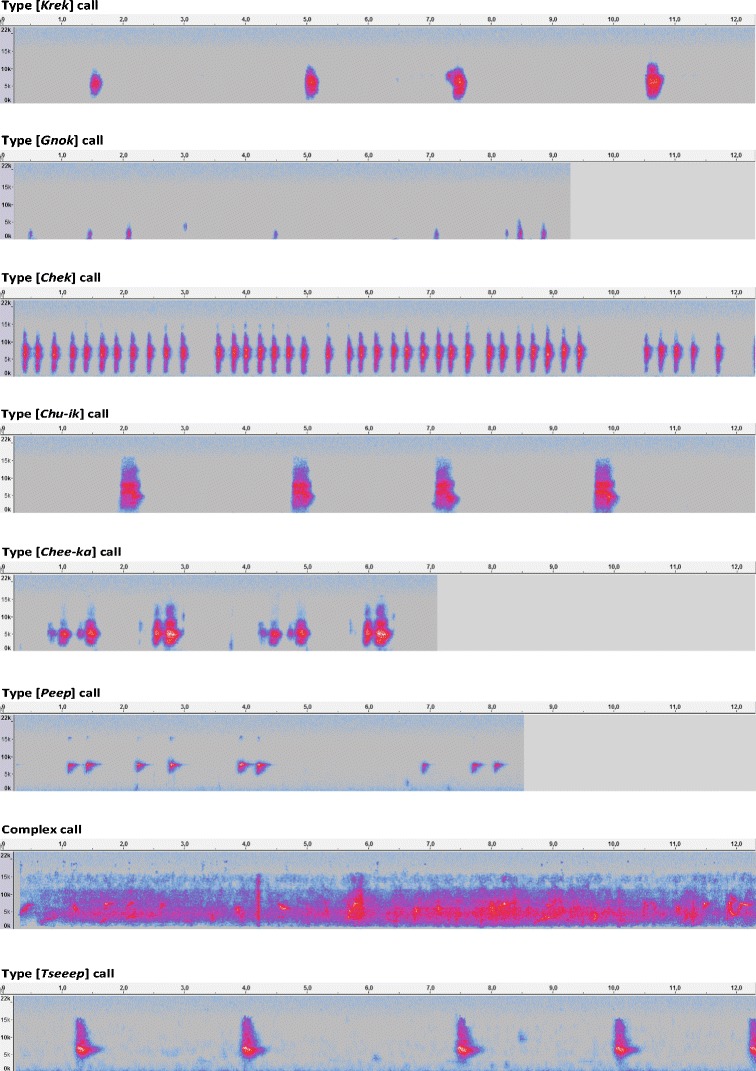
Sonograms of White-breasted Thrasher’s (*Ramphocinclus brachyurus brachyurus*) vocal repertoire (created with Audacity)
